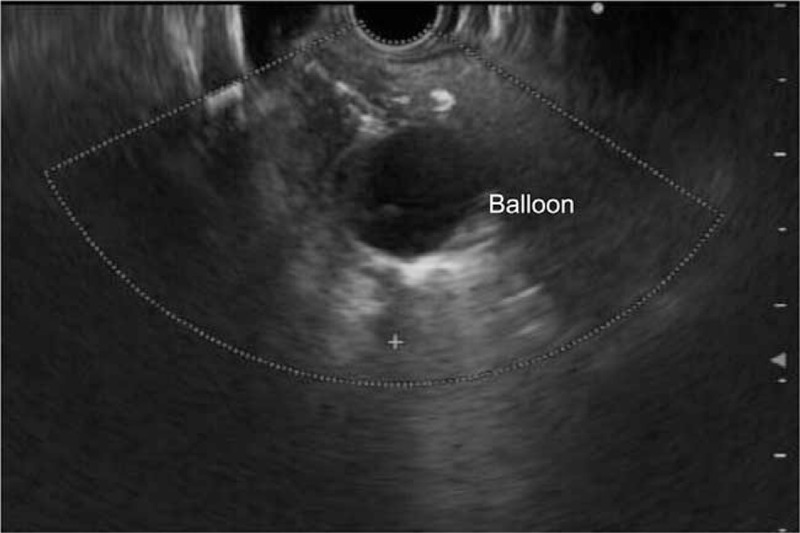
Endosonographic image. The inflated balloon inside the narrow fistula tract.
